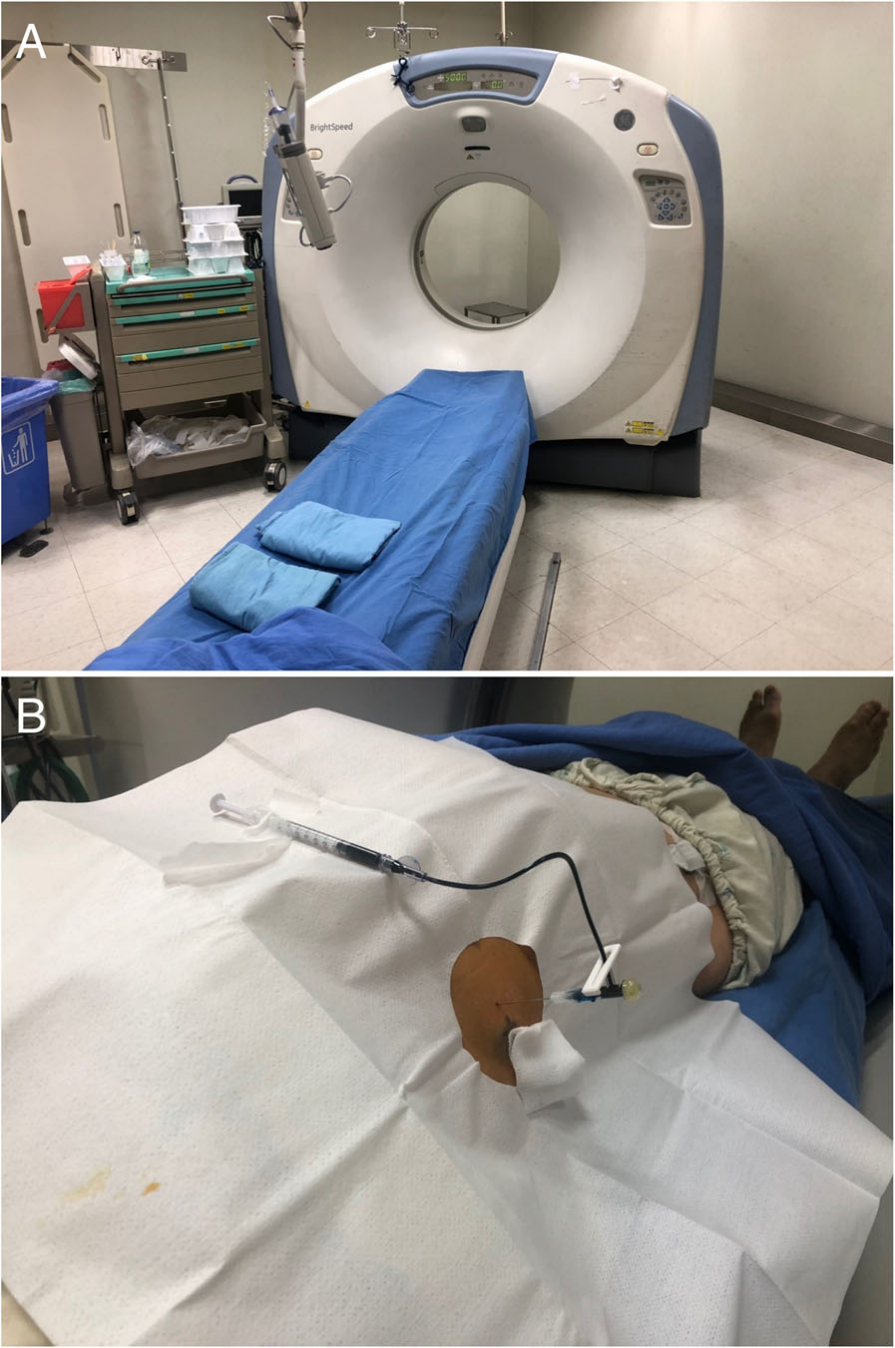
a Interventional radiology suite equipped with a 16-slice CT scanner (GE BrightSpeed; GE Healthcare, Milwaukee, WI, USA). b PBV dye injection following needle localization
